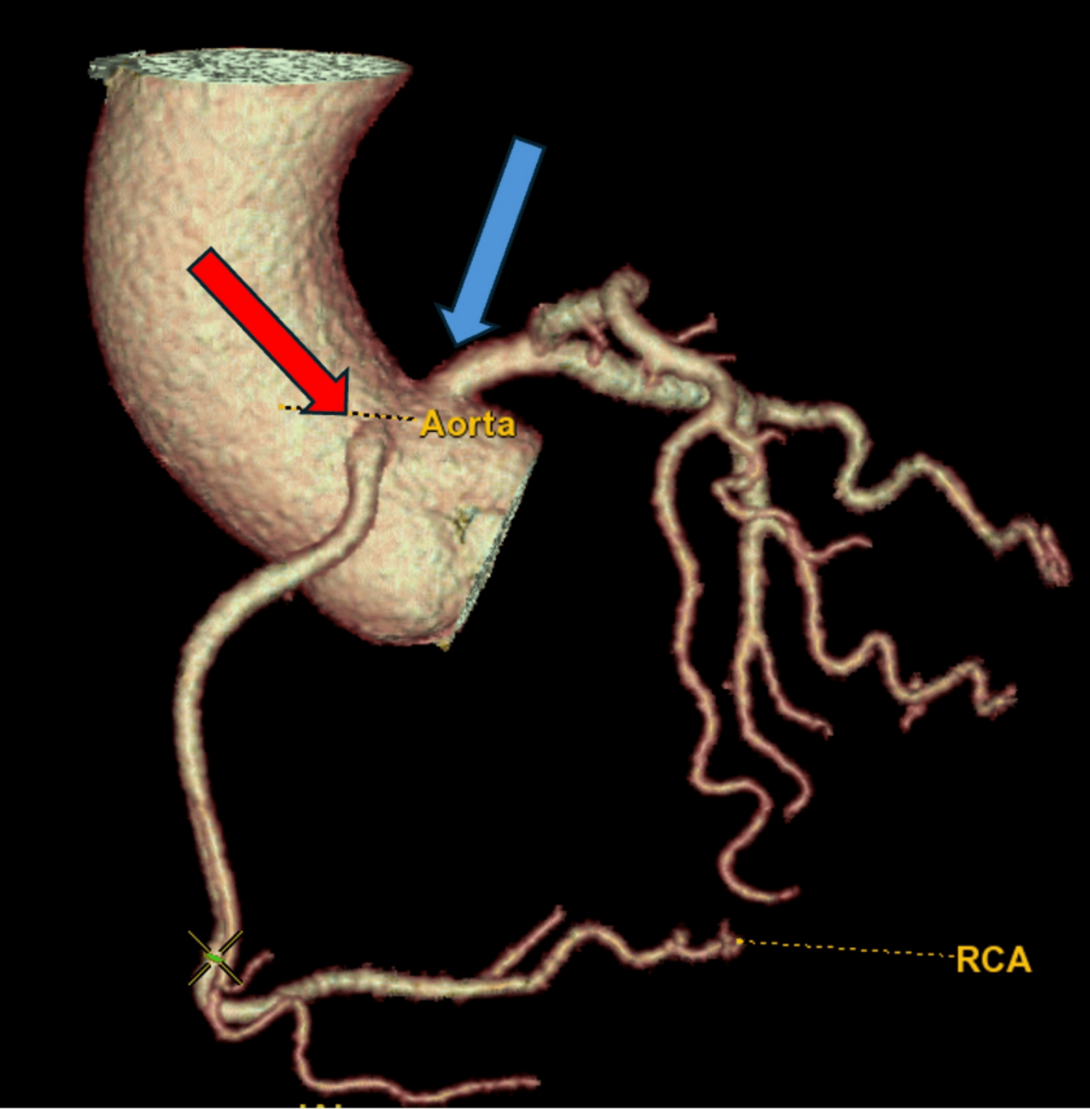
Coronary computed tomography angiography with a 3D-rendered image of the anomalous right coronary artery, originating from the left coronary cusp, anterior to the origin of the left main artery, and coursing anteriorly between the ascending aorta and the main pulmonary artery. The red arrow indicates the anomalous right coronary artery. The blue arrow indicates the left main artery.
